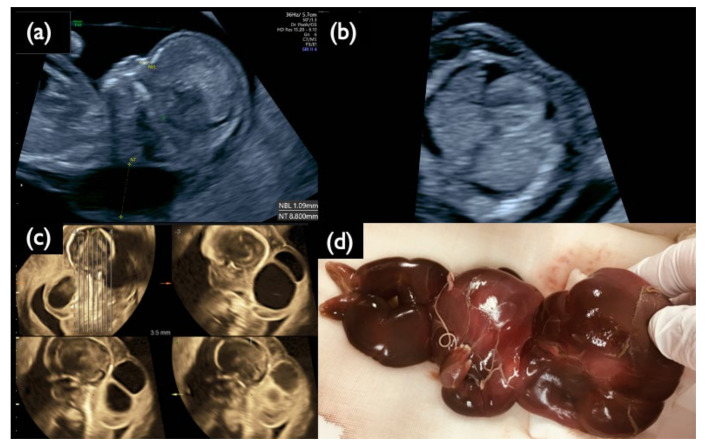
Sonographic findings at 11^+6^ weeks, and 17 weeks, and IUFD fetus after delivery in Case FP1. (a) Mid-sagittal section of the fetal head. Nuchal translucency (NT) of 8.8 mm and a small nasal bone are demonstrated. (b) Horizontal section of the thoracic area. Pleural effusion is visualized bilaterally. (c) 3D tomographic ultrasound image in the sagittal section with a coronal guide section (left upper). General edema and large cystic hygromas are shown. (d) Picture of the dead fetus on delivery at 20 weeks. The fetus died in utero at 19 weeks of gestation.
